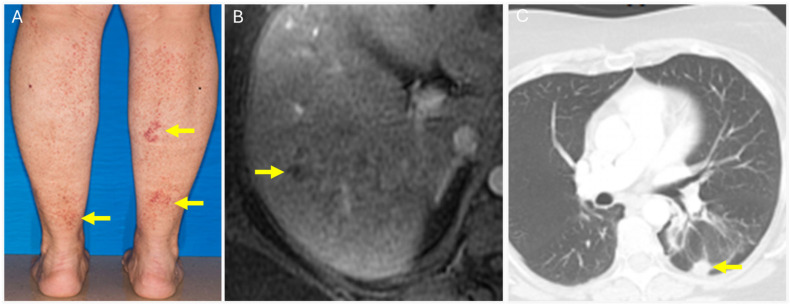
A 51-year-old female with a history of IgG kappa monoclonal gammopathy presented with persistent and increasingly tender nodules of the posterior calves (A, yellow arrows). Skin biopsy showed NXG. MR abdomen (B, yellow arrow) revealed hepatic infiltration with innumerable non-enhancing tiny nodules, and liver biopsy showed NXG. Chest CT (C, yellow arrow) found a left lower lobe nodule, and lung biopsy showed NXG.
